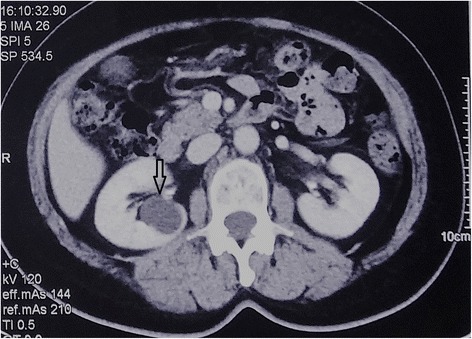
CT image showing renal cyst (arrow) in Patients B24
